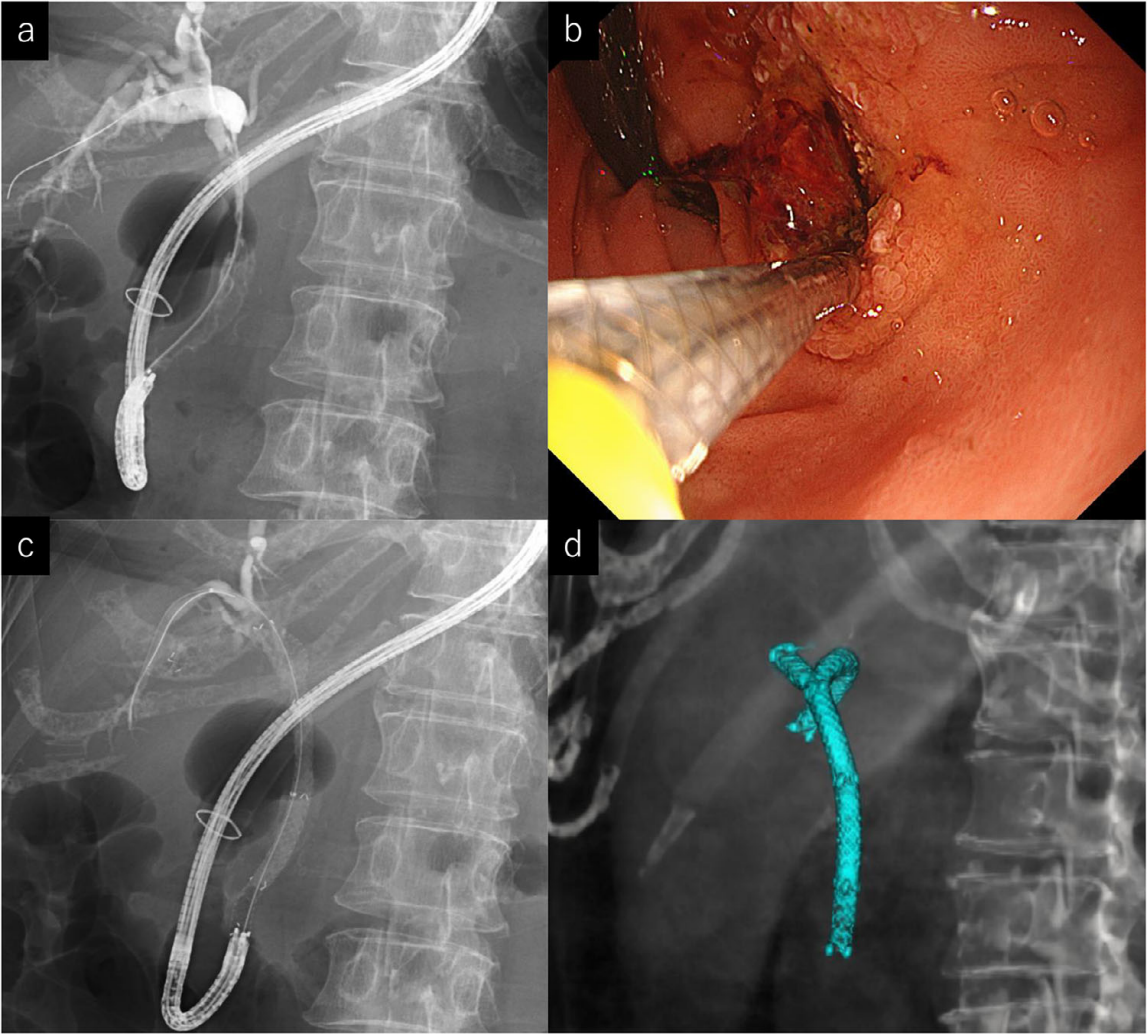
(a) Using the overtube‐assisted ultrathin endoscope approach, cholangiography demonstrated hilar biliary stricture. (b, c) With retroflexion of the ultrathin endoscope, MHSEMSs were deployed into the right anterior and posterior segmental bile ducts using the partial stent‐in‐stent technique. MHSEMSs: multi‐hole self‐expandable metal stents. (d) Three‐dimensional reconstruction of the stents based on CT images after stent placement. CT, computed tomography.
